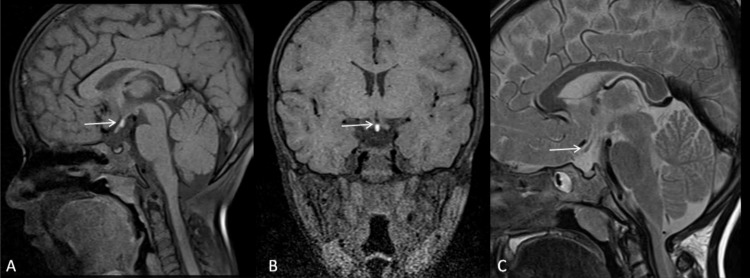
Sagittal (A) and coronal T1 (B), sagittal T2 (C): weighted MR images of pituitary fossa shows non-visualization of infundibulum, absence of bright spot of posterior pituitary at the normal site, ectopic posterior pituitary near the median eminence (marked by white arrow).
